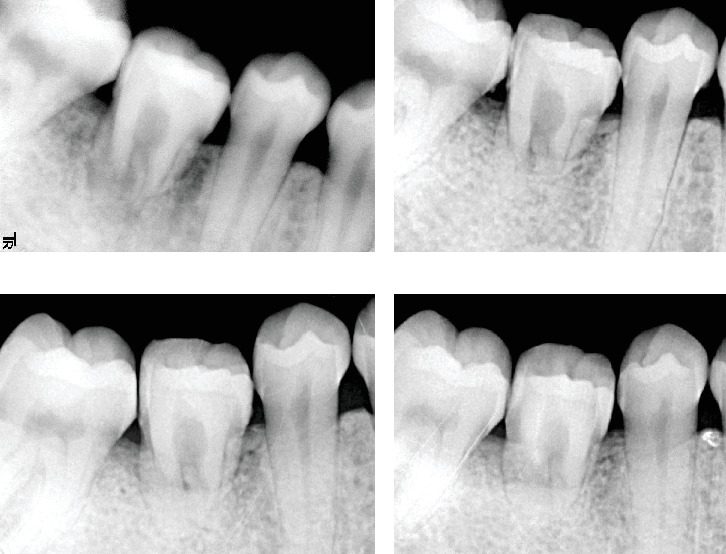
(a) A radiograph taken at 1 year after the transplantation. (b) A radiograph taken at 6 years after the transplantation. (c) A radiograph taken at 9 years after the transplantation. (d) A radiograph taken at 11 years after the transplantation.
